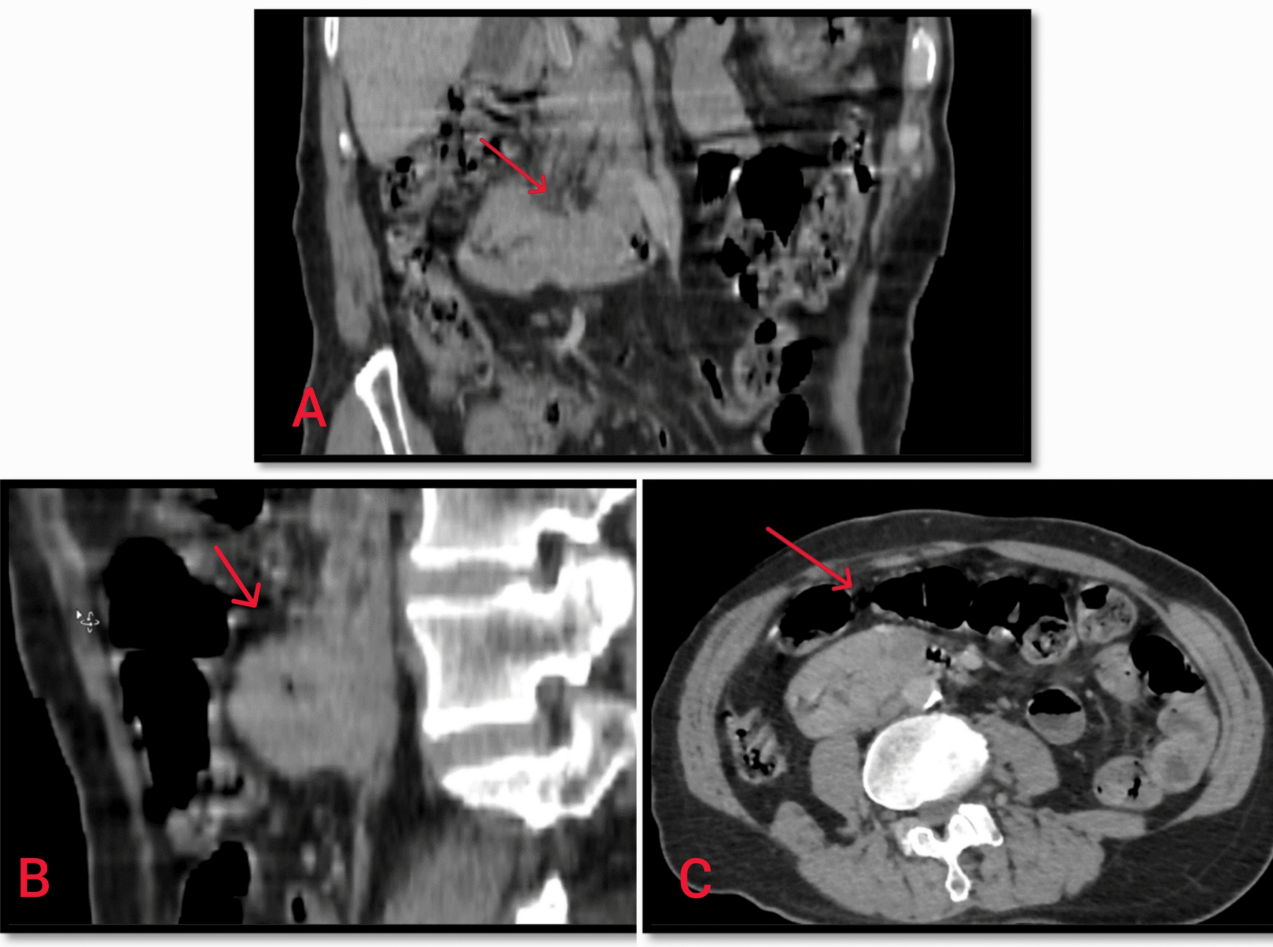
Contrast-enhanced abdominal CT with multiplanar reconstructions. A regular, circumferential, symmetric prestensotic endoluminal duodenal wall thickening is centered at the D2–D3 junction (red arrow), without suspicious locoregional lymphadenopathy and without CT signs of upstream obstruction. (A) Coronal multiplanar reconstruction (MPR): circumferential wall thickening at the D2-D3 junction (red arrow). (B) Sagittal MPR: segmental prestensotic endoluminal thickening involving the D2-D3 junction (red arrow). (C) Axial CT: confirmation of symmetric circumferential thickening at the D2-D3 junction (red arrow).
